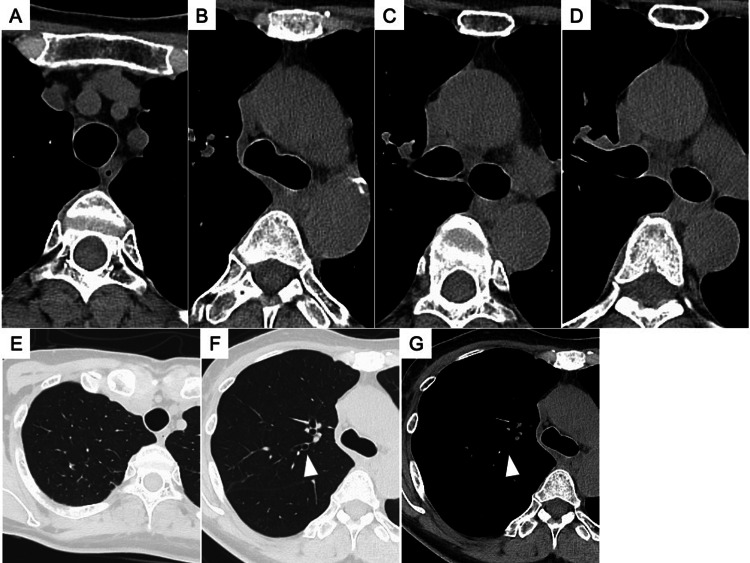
Chest computed tomography findings at the end of anti-tuberculosis treatment. Chest computed tomography at the end of anti-tuberculosis treatment shows that the circumferential wall thickening in the trachea (A), carina (B), right main bronchus (C), and right upper lobe bronchus (D) has improved. Granular and nodular shadows in the right S^2^ of the lung and a nodular shadow in the orifice of the right B^2^ (white arrowhead) have also improved (E-G).
